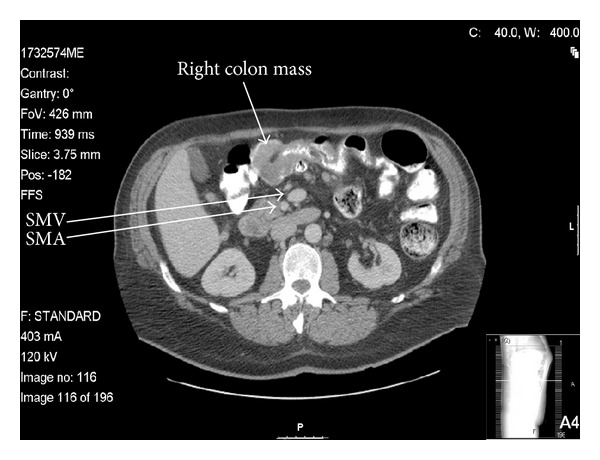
CT showing SMA dorsal to SMV.
